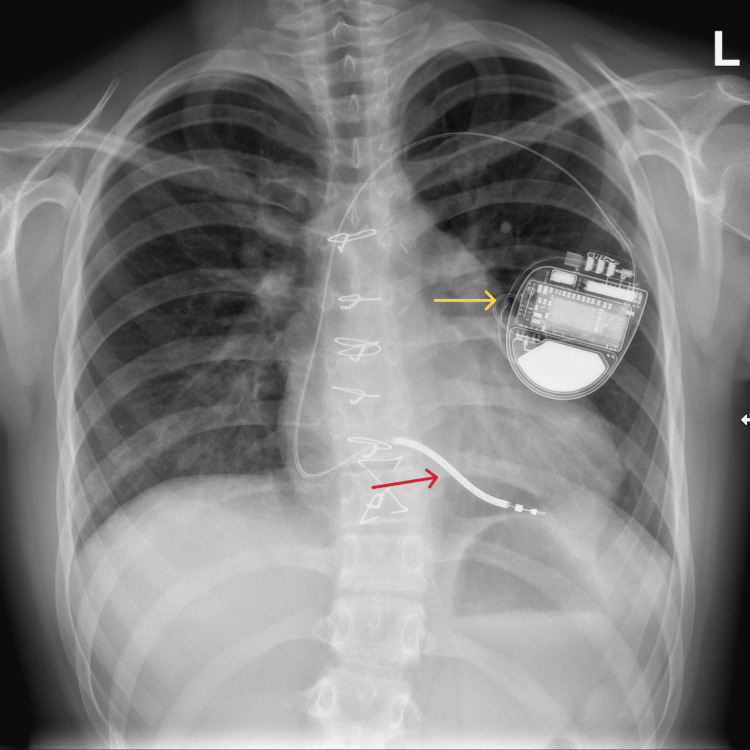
Chest X-ray demonstrating a left-sided single-chamber ICD Chest radiograph demonstrating a left-sided transvenous implantable cardioverter-defibrillator (ICD) with the pulse generator positioned in the left infraclavicular region (yellow arrow) and a single transvenous lead, with the distal tip positioned in the right ventricular apex and the defibrillation coil visible (red arrow).
